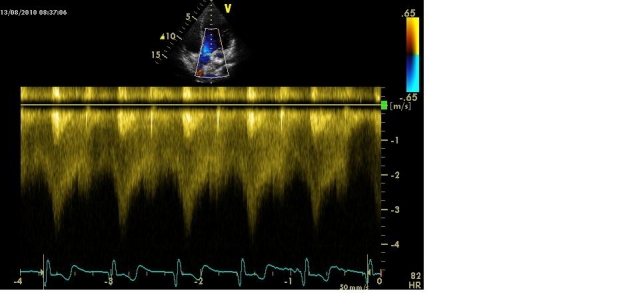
Coronary fistula between coronary left sinus and right atrium. Doppler evaluation at the tortuous ductus (described above) reveals a continuous flow with increased velocity.
